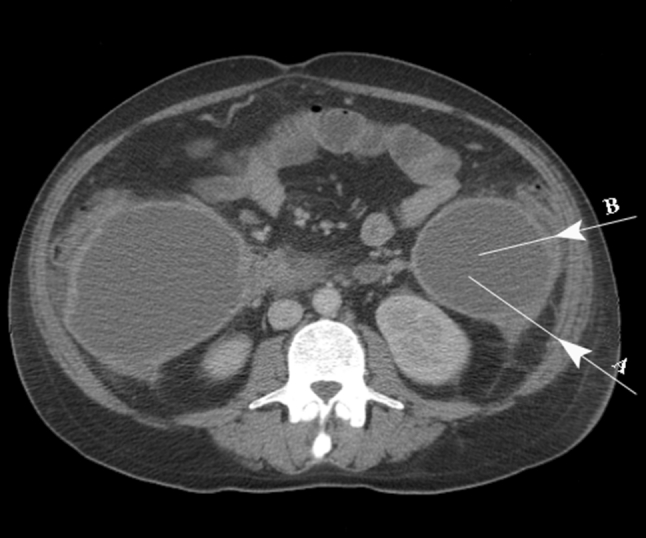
Computed tomography image indicating the appropriate (*arrow A*) and incorrect (*arrow B*) direction for placement of the drainage catheter. Choosing the latter route may result in traversing the peritoneal recessus and leakage of the necrotic material into the peritoneal cavity
